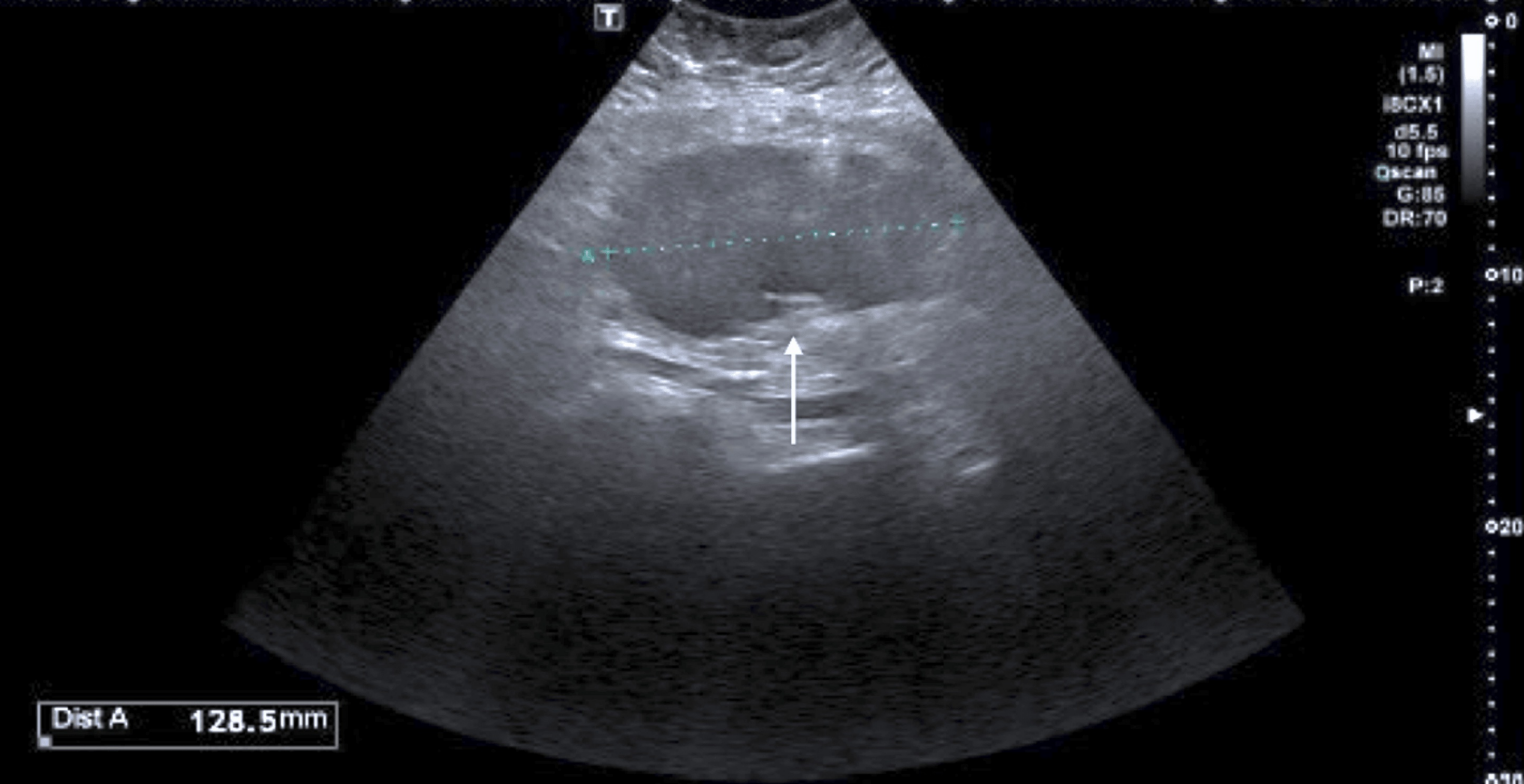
Ovarian ultrasound echography The first ultrasound echography (September 2019) detected left ovarian metastasis with irregular margins (128.5 mm).
